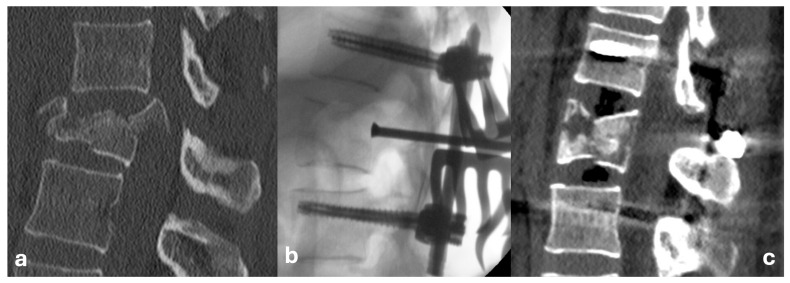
Combined reduction technique: (a) CT sagittal plane projection (bone window) of an L1 AO Type A4 fracture; (b) Perioperative C-arm guided lateral projection radiograph of a direct fracture reduction using an impactor; (c) CT sagittal plane projection showing final lordosis achieved through ligamentotaxis.
